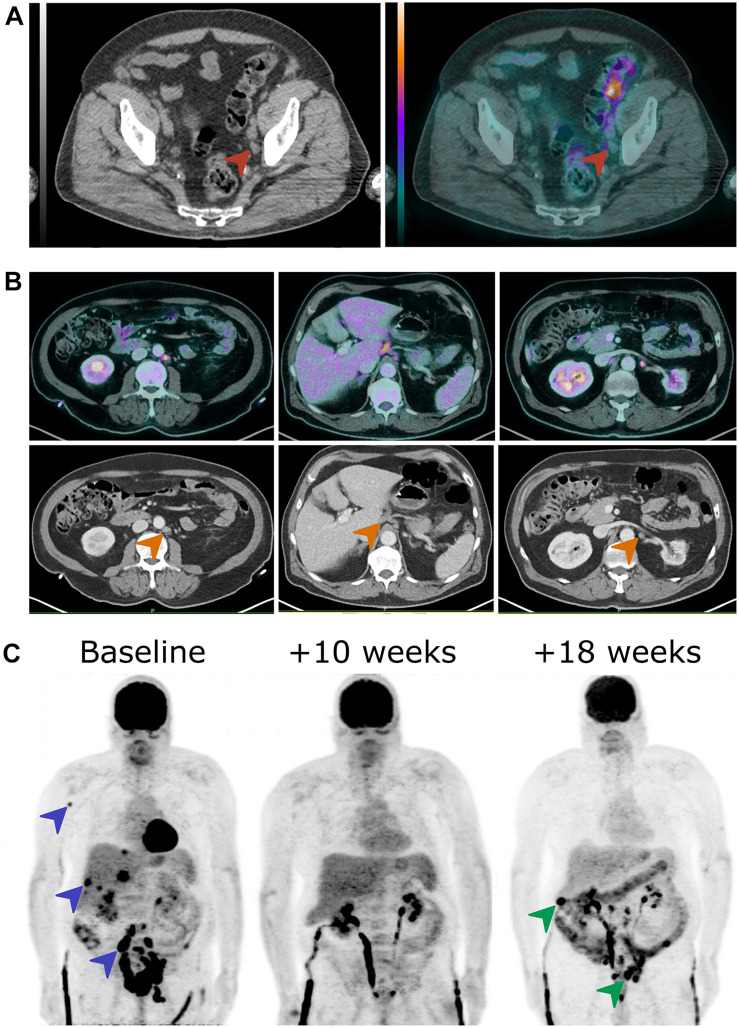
18F-FDG PET/CT images of three patients at different time points in the course of MIBC. (A) A 76-year-old man presented with an enlarged external iliac lymph node at initial staging without any 18F-FDG uptake (red arrowheads). The N0 status was confirmed by pathological examination of pelvic lymph node dissection sample. (B) A 67-year-old man was oligometastatic with one enlarged latero-aortic lymph node on CT at initial staging and 18F-FDG PET/CT revealed several other retroperitoneal lymph node metastases (orange arrowheads). (C) In a 62-year-old man who presented MIBC with osseous, hepatic, and nodal metastases at diagnosis (blue arrowheads), 18F-FDG PET/CT demonstrated complete metabolic response after three cycles of chemotherapy and then a locoregional and hepatic relapse after the fifth chemotherapy cycle (green arrowheads). 18F-FDG PET/CT, 2-deoxy-2-(18F)fluoro-D-glucose positron emission tomography/computed tomography; MIBC, muscle invasive bladder cancer.
